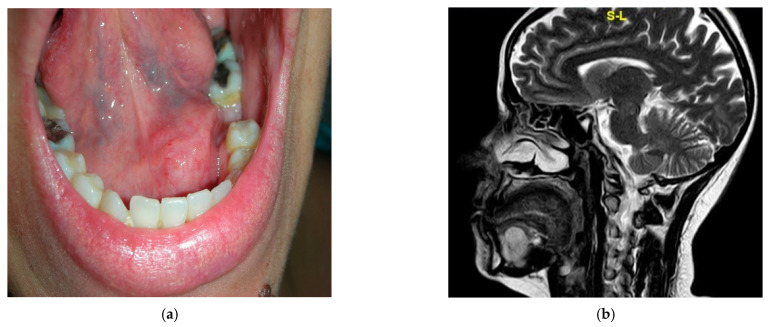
(a) Multiplanar MRI performed on the patient. (b) Preoperative images of our patient’s mass.
